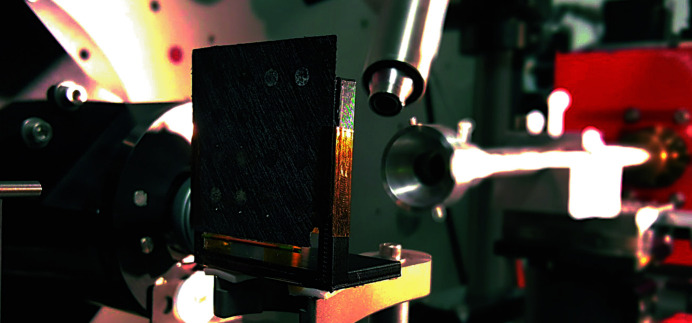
The combinatorial library mounted on a 3D-printed bracket in front of the X-ray beam. The array is mounted on the goniometer which allows measurement access to all deposition sites.
